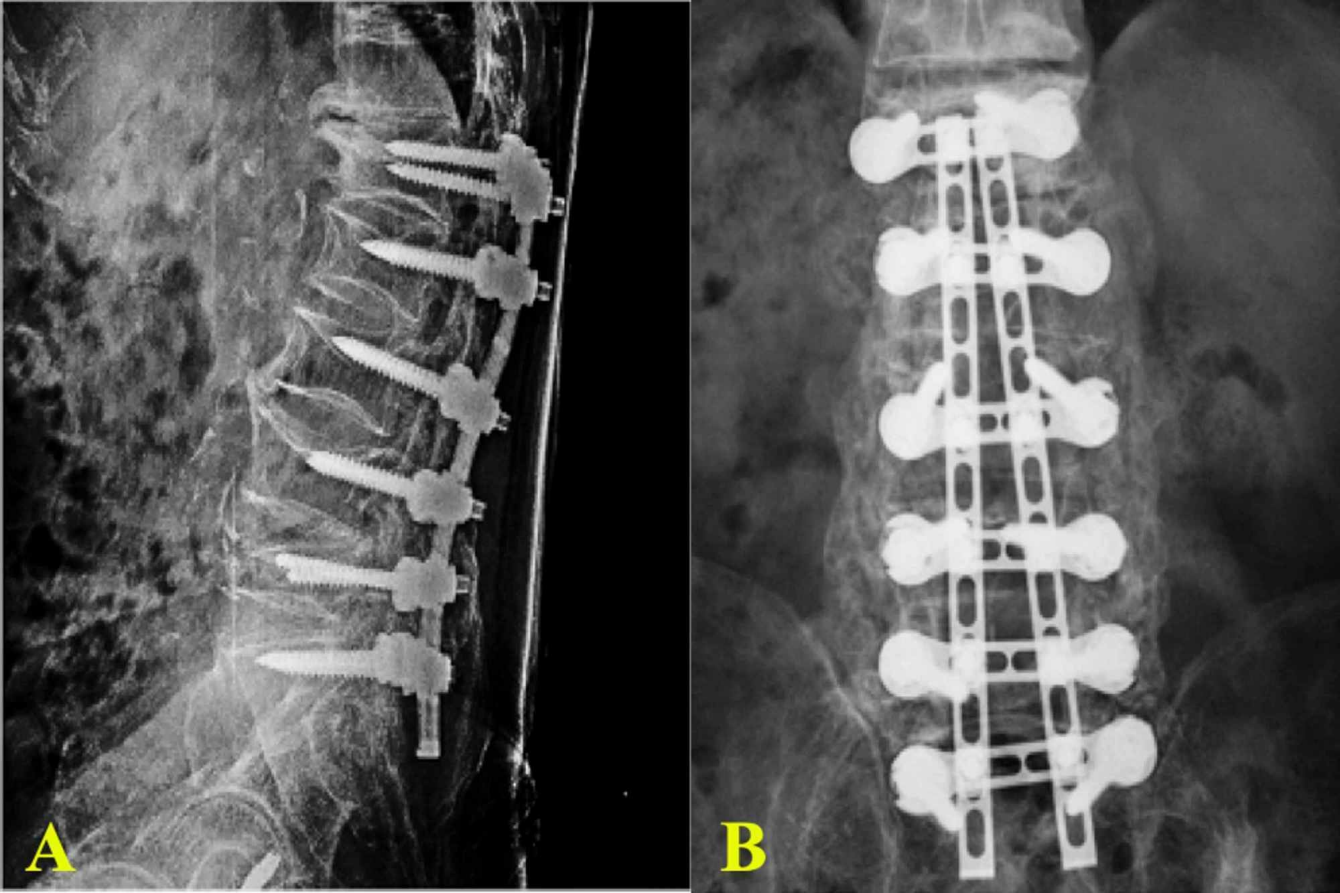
Postoperative X-ray images (AP view (A), lateral view (B)) that show lumbar compression fractures and the instrumented fusion of the L1-S1 levels using the UNIMAX system
